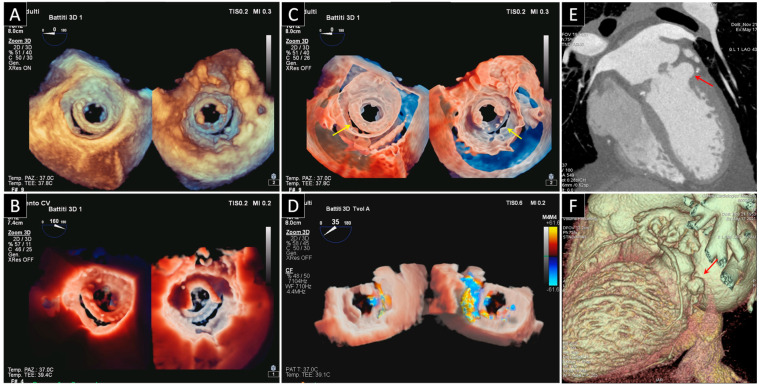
Bioprosthetic mitral valve detachment plus annulus pseudoaneurysm. (A) Standard 3D TEE rendering of prosthetic mitral valve from left atrial (surgeon’s view) and ventricular perspective demonstrating detachment from 6 to 9 o’clock due to prosthesis dehiscence. (B) Transillumination rendering technique with virtual light source highlighting the localization of the detachment. (C) 3D transparency technique more clearly delineating the borders and edges of the paravalvular defect (yellow arrows). (D) 3D transparency feature merged with color clearly showing the presence of a severe regurgitation through the area of periprosthetic detachment. (E) CT imaging revealing a mitral prosthetic annulus pseudoaneurysm (red arrow) at the ventricular posterolateral wall just under the sewing ring near the detachment of the prosthetic valve in the same patient (F) 3D volumetric CT reconstruction of the pseudoaneurysm (red arrow).
